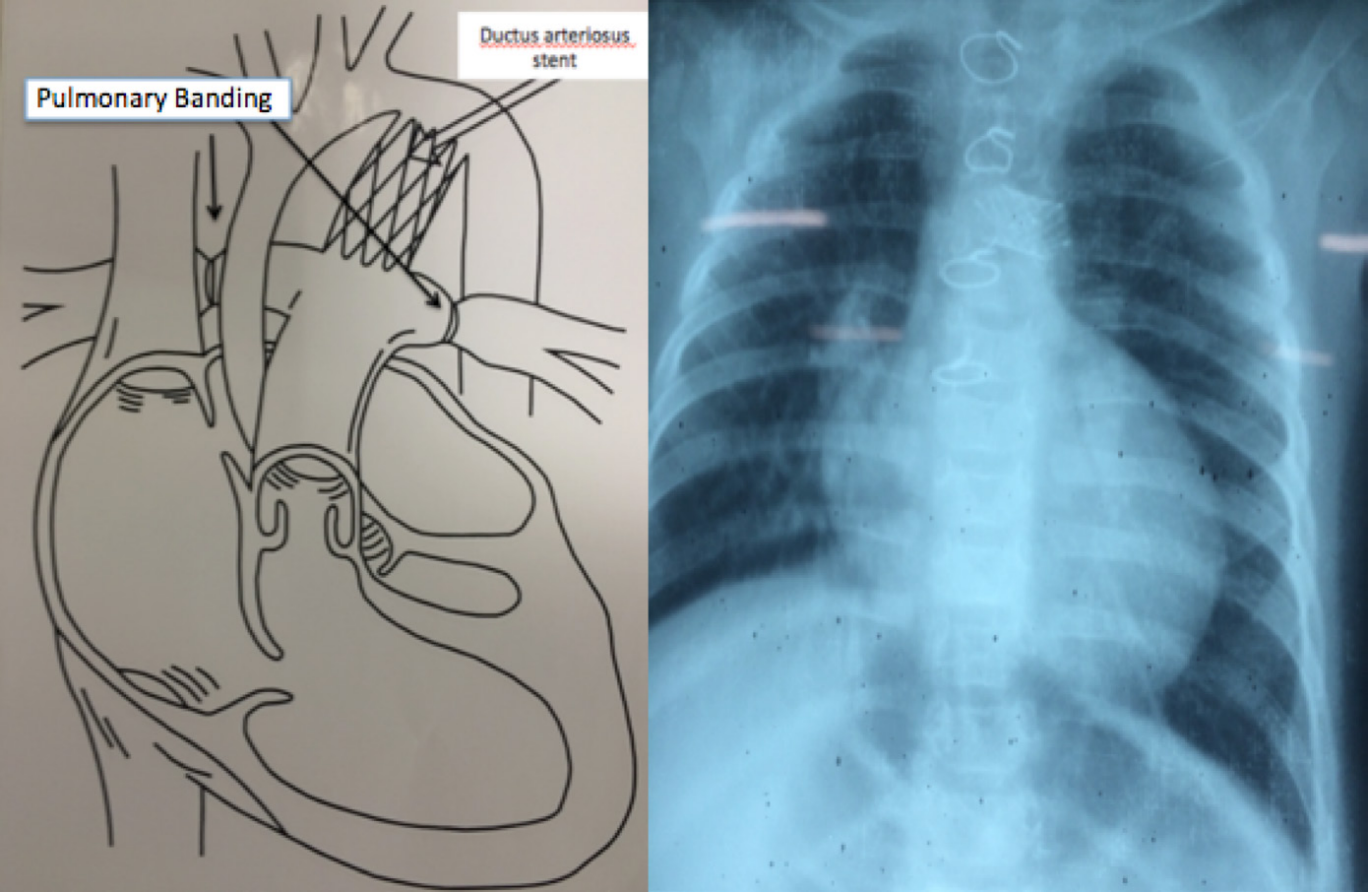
Final surgical and radiographic aspect of the hybrid procedure.
